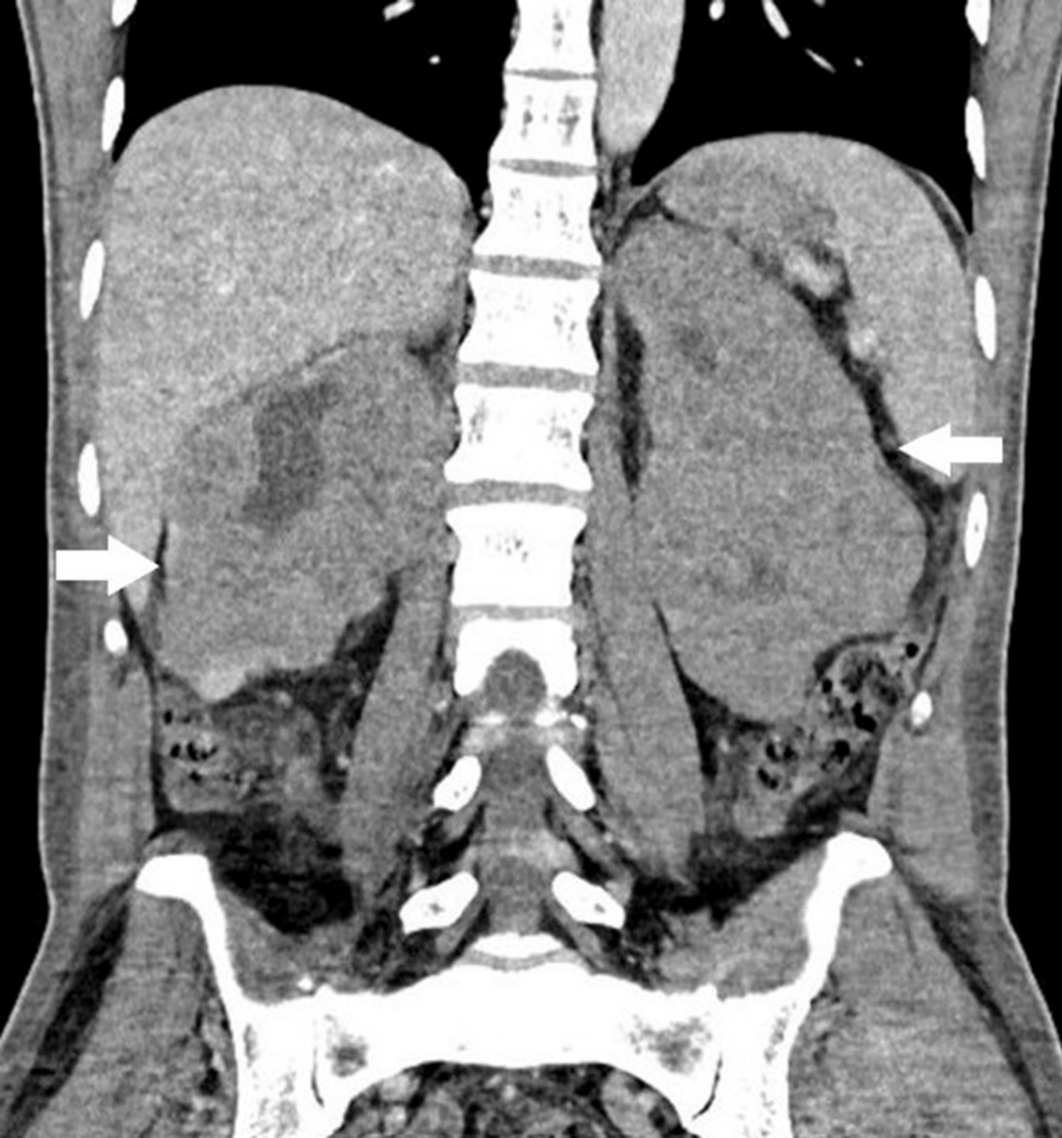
Coronal abdominal CT scan showing bilateral nephromegaly with hypodense parenchymal infiltrates, without extracapsular extension, compatible with renal lymphoma.
